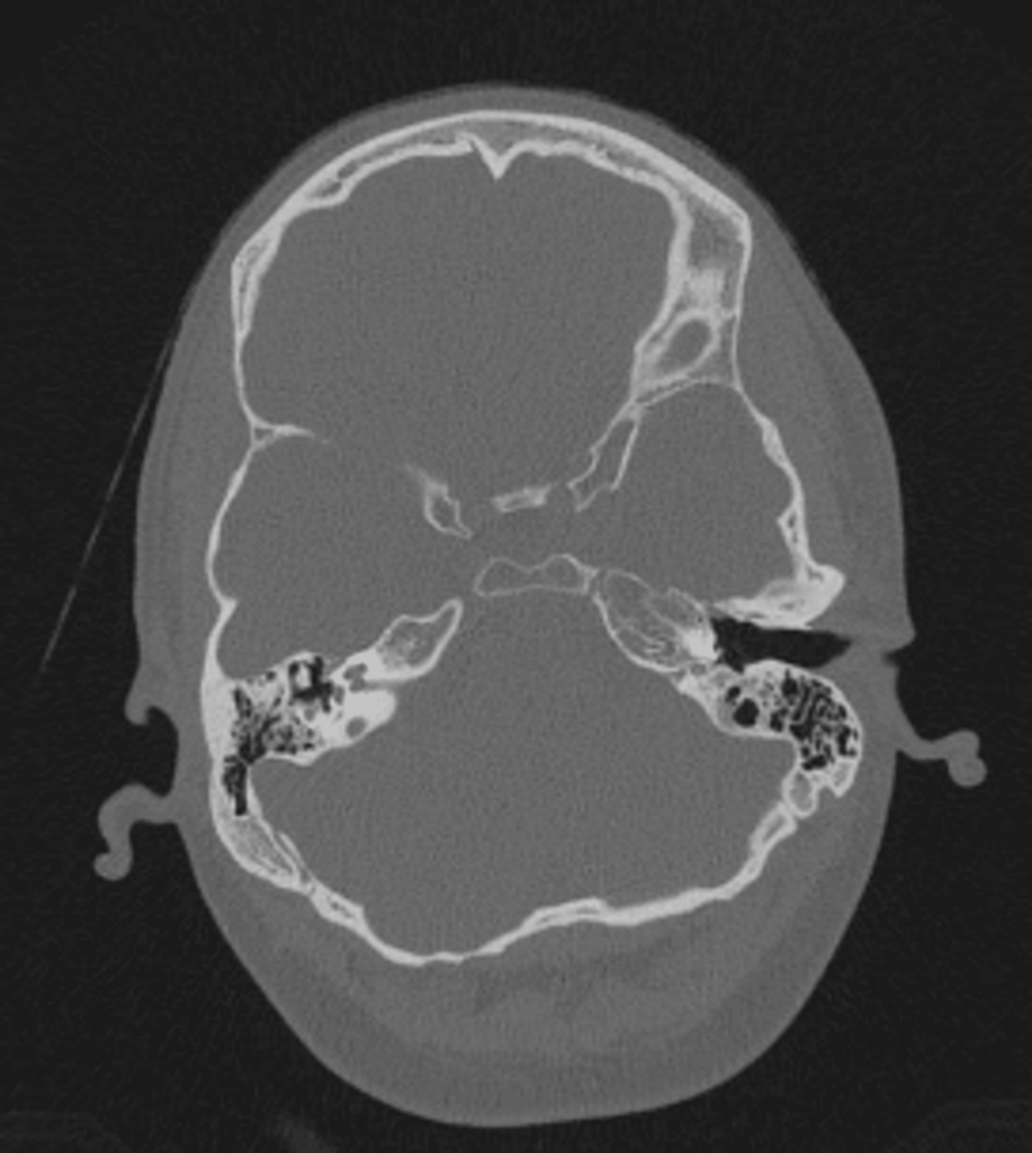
Axial CT in bone window indicating bilateral endolymphatic hydrops. Source: Gerstenmaier J. Ménière disease. Radiopaedia.org. (Accessed on 09 May 2025). rID: 24268 (https://doi.org/10.53347/rID-24268). Used under the Creative Commons Attribution-NonCommercial-ShareAlike license.
